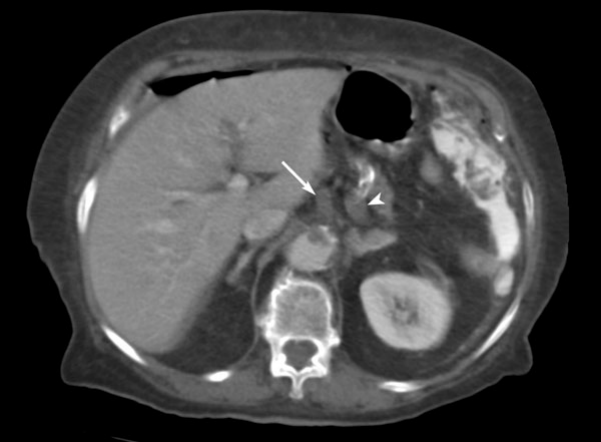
Contrast-enhanced spiral CT shows thrombus in the trunk of the celiac artery (arrow) protruding in the lumen of the abdominal aorta, and extending into the splenic artery (arrowhead).
